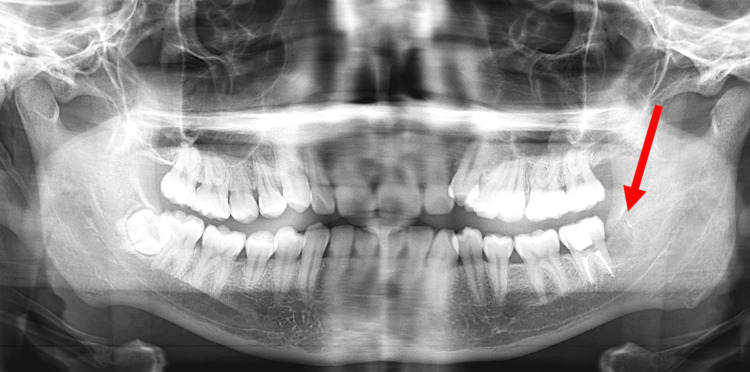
Panoramic radiograph showing the extracted site of the lower-left third molar.
